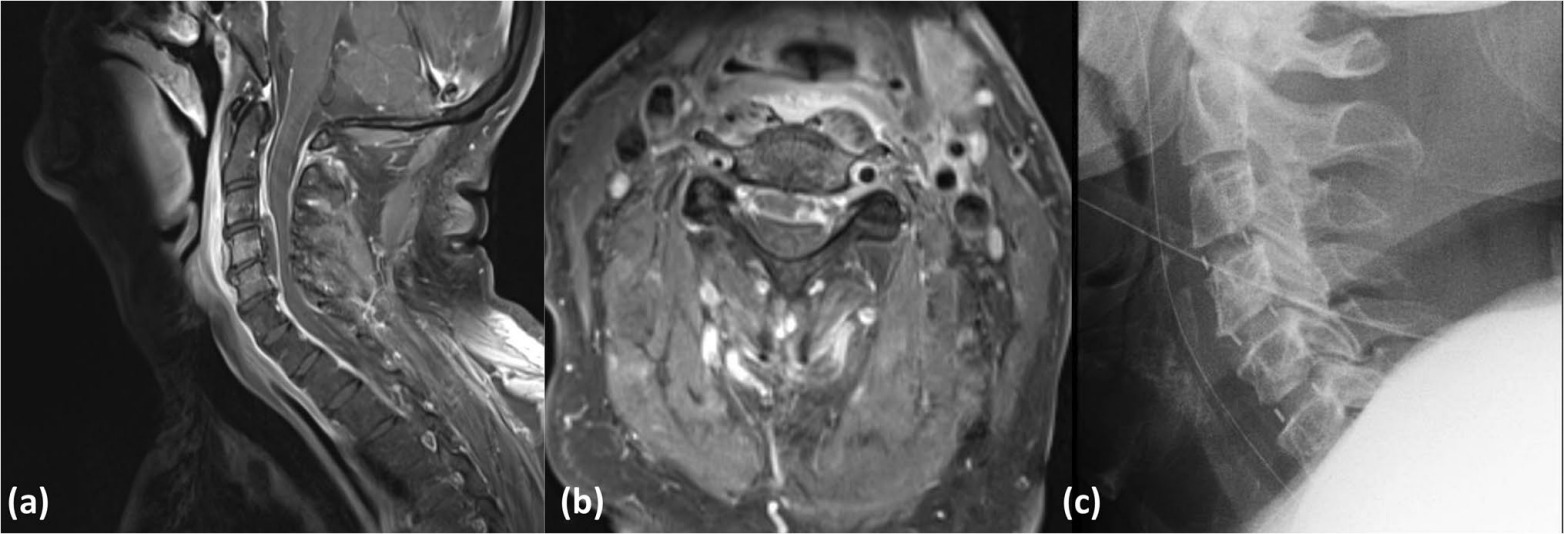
A 57-year-old man presented with progressive tetraparesis and severe sepsis. Sagittal and axial MRI (T1-weighted with gadolinium) shows cervical spondylodiscitis and an intraspinal longitudinal abscess with compression of the spinal cord (a, b). Emergency surgery was performed including ventral discectomy, decompression of the epidural abscess, and insertion of intervertebral cages at 3 cervical levels (c). During ICU treatment and antibiotic therapy, the tetraparesis resolved completely, and the patient was discharged to rehabilitation
